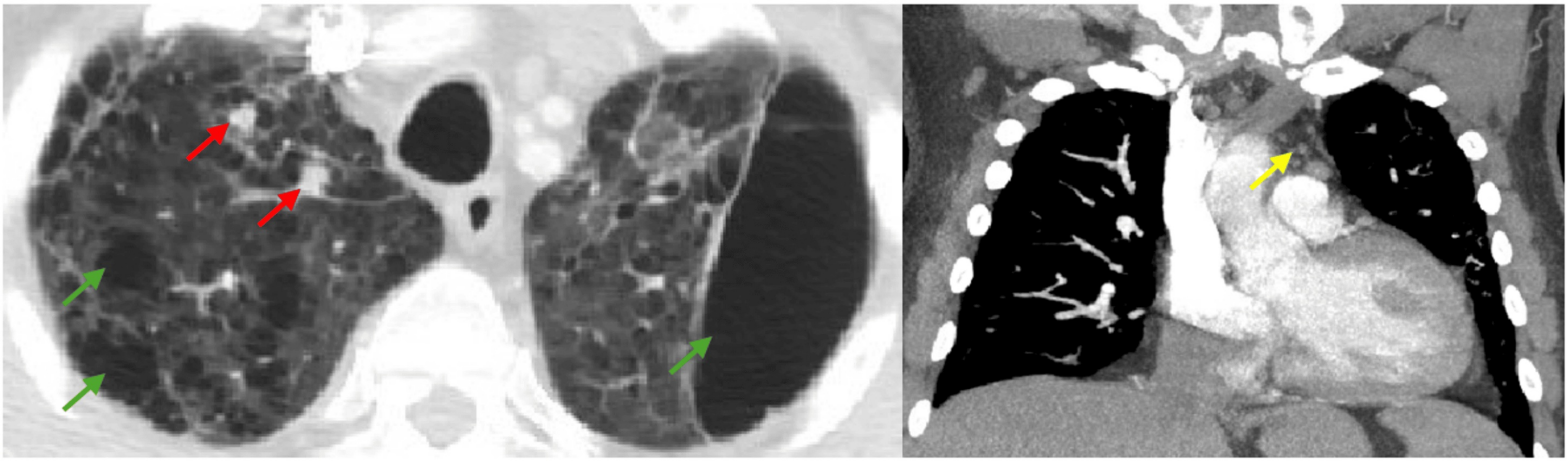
Images from the chest CT showing markedly emphysematous lungs (green arrows), pulmonary nodules (red arrows), and mediastinal lymphadenopathy (yellow arrow).
